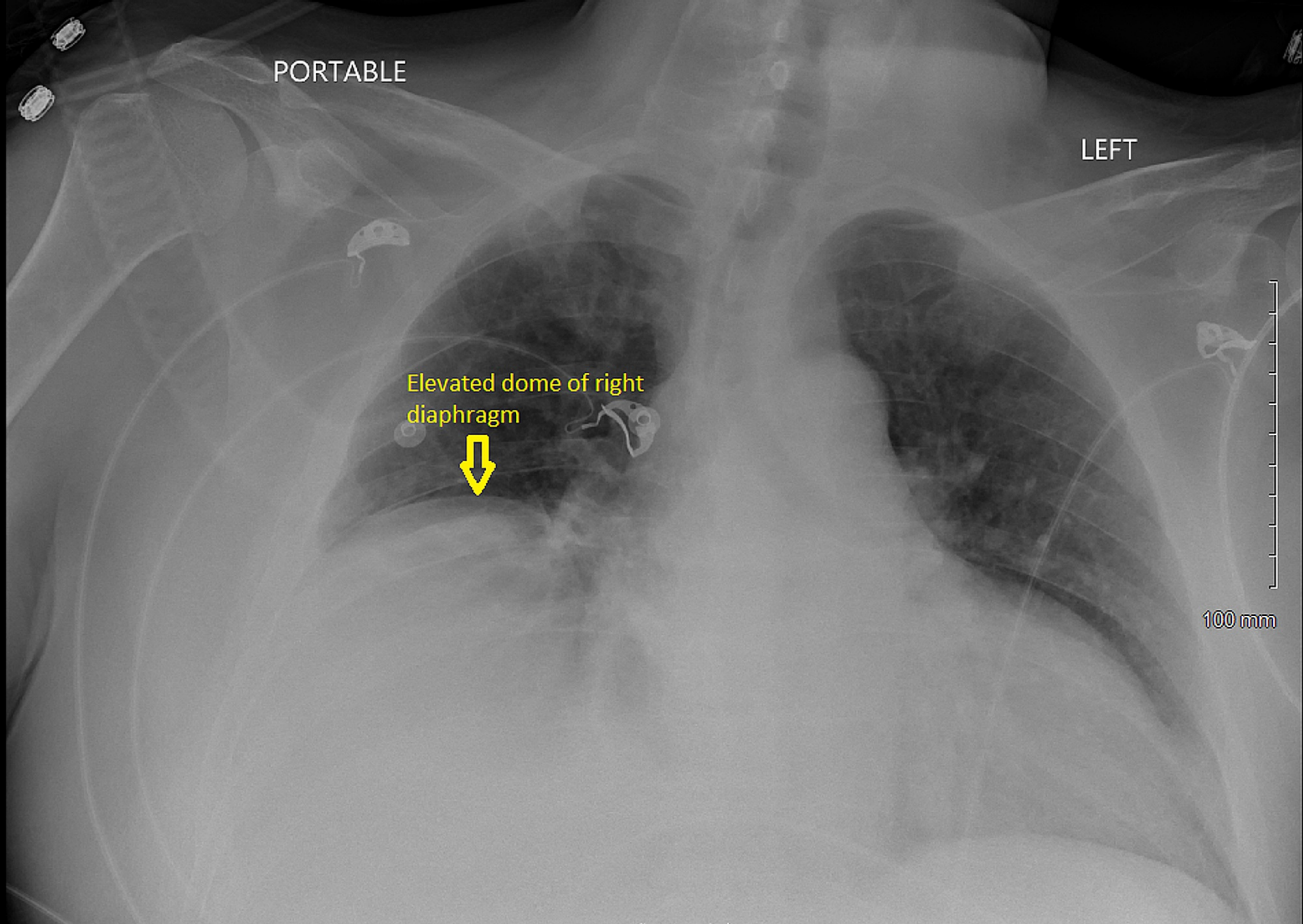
Anteroposterior chest X-ray depicting the elevation of right-sided hemidiaphragm post stroke.
